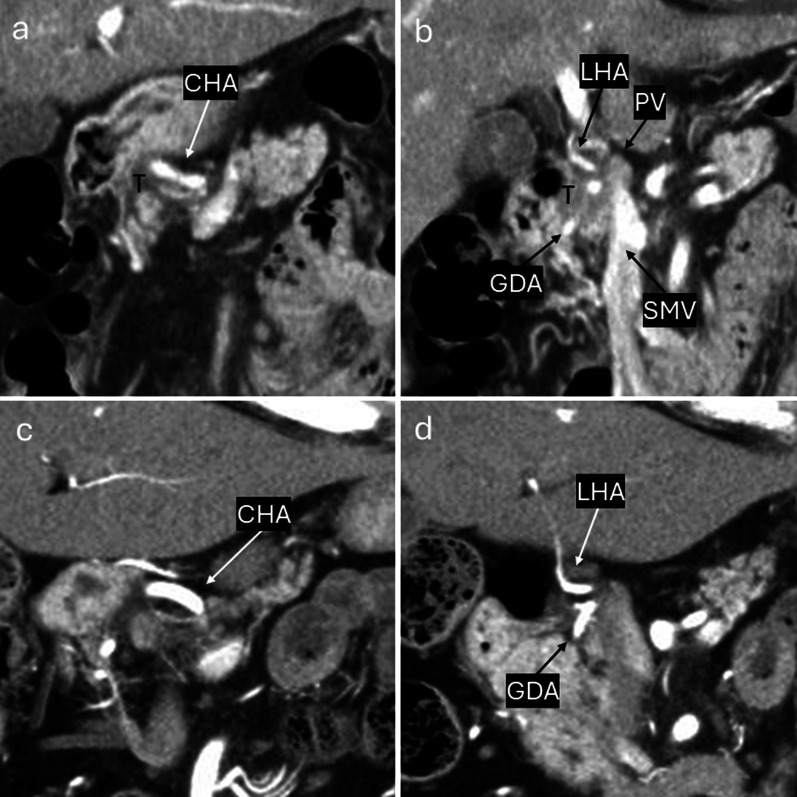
Multidetector row computed tomography (MDCT) scans of a pancreatic adenosquamous carcinoma. a, b Contrast-enhanced MDCT scans before conversion-intended chemotherapy. The tumor had invaded the common hepatic, left hepatic, and gastroduodenal arteries and contacted the superior mesenteric and portal veins. c, d Contrast-enhanced MDCT scans after conversion-intended chemotherapy. The margin of the common and left hepatic arteries became distinct, and clear fat planes around these arteries were observed. GDA, gastroduodenal artery
